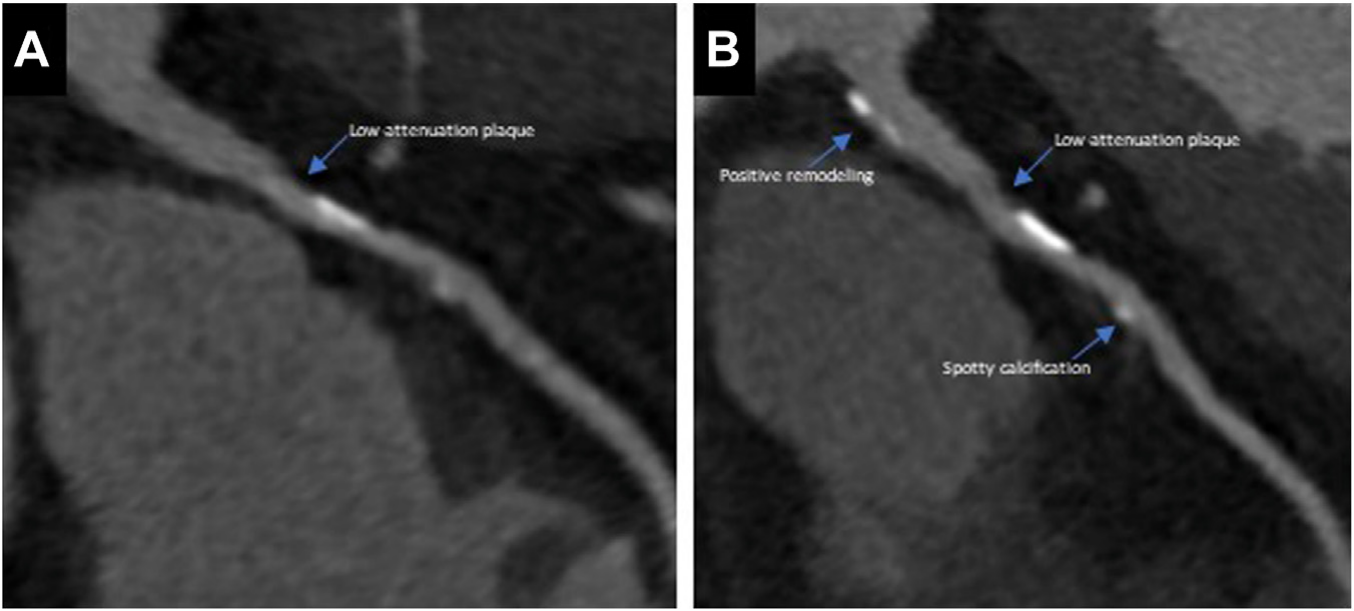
Progression of coronary artery disease and high-risk plaque features on coronary computed tomography angiography (CCTA). An example of a patient with plaque progression and an increase in high-risk plaque features shown on serial CCTA with (A) baseline and (B) follow-up CCTA of the proximal left anterior descending artery.
